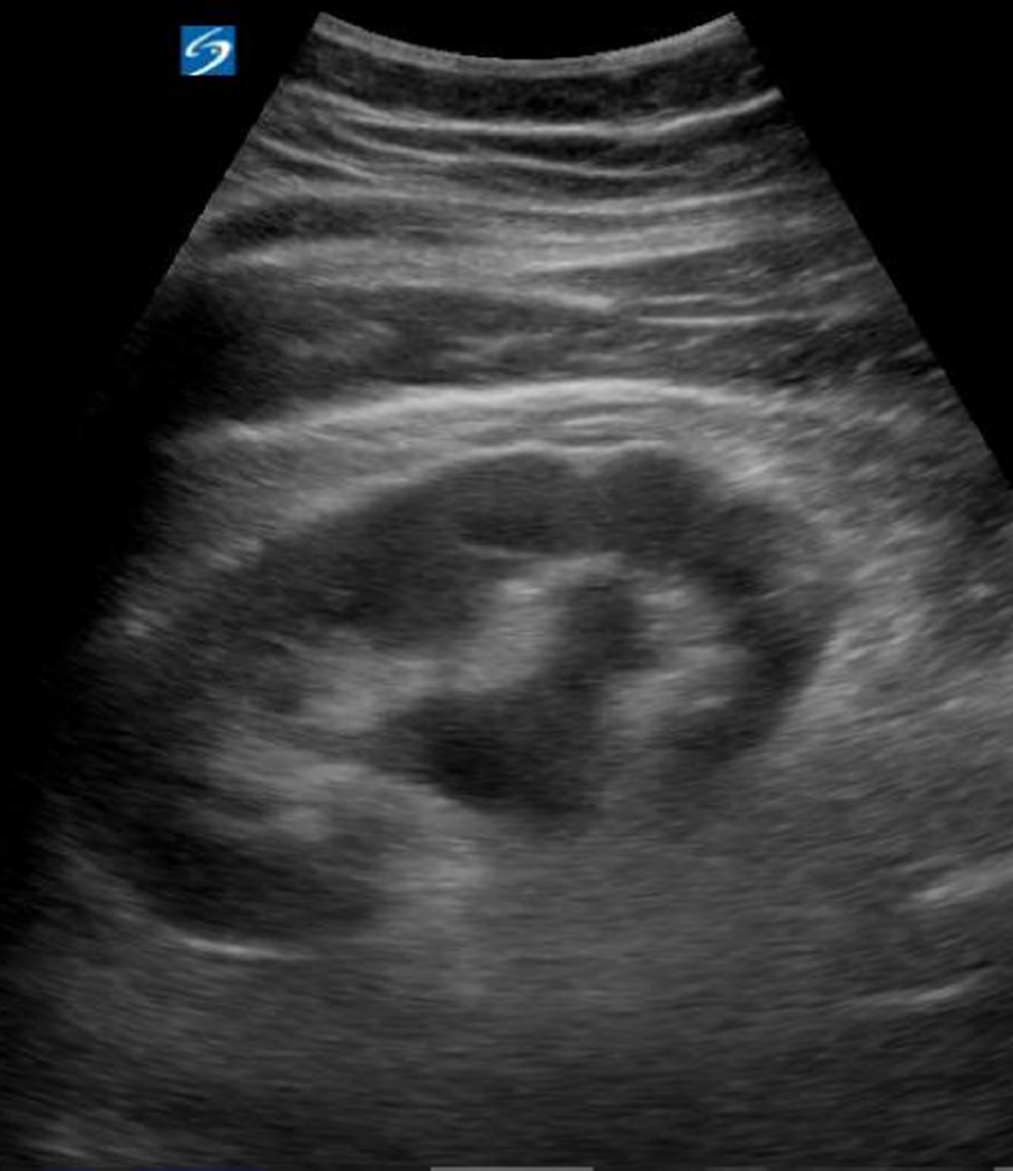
Point of care ultrasound (POCUS) demonstrating hydronephrosis.
